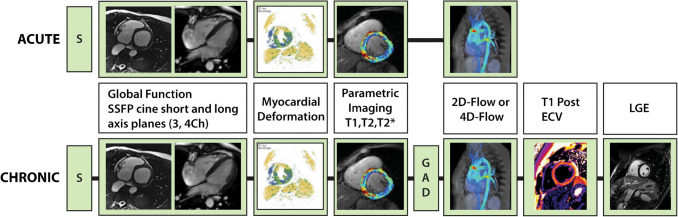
Cardiac MRI protocol for evaluation of cardiotoxicity in pediatric cancer patients. 2D, two dimensional; 4D, four dimensional; Ch, chamber; ECV, extracellular volume; GAD, gadolinium-based contrast; LGE, late gadolinium enhancement; SSFP, steady-state free precession
